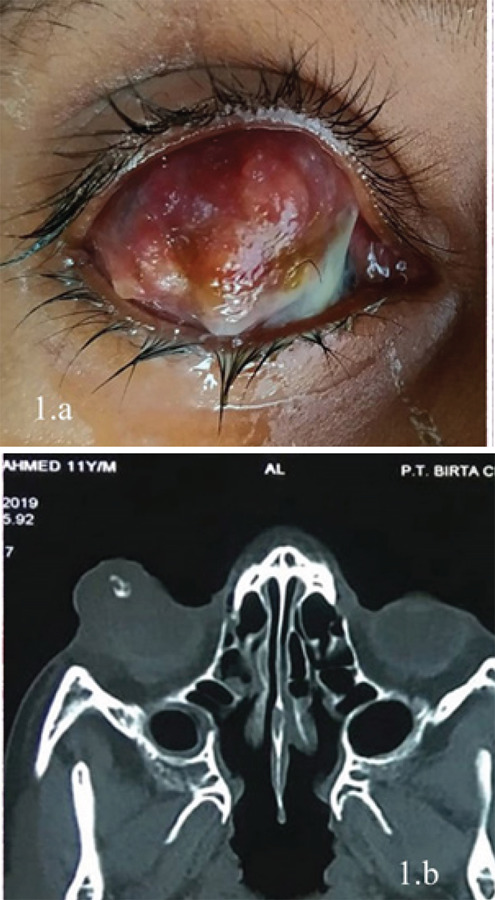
a. Clinical photograph demonstrating subconjunctival mass. b. CT-Scan image of orbit demonstrating hyperdense focus with surrounding thickening and irregular wall of the globe.
